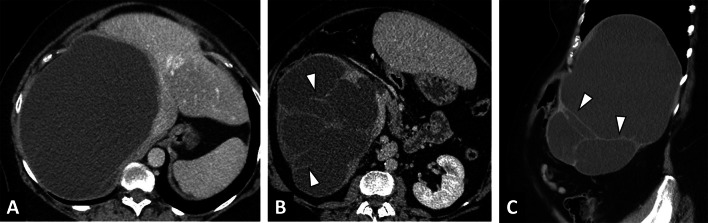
Differential diagnosis of cystic echinococcosis lesion in a 64-year-old woman. A CT image in the axial plane at the portal phase showing a large right liver cyst. B Same examination, lower slices. Internal enhanced septa. C CT image in the sagittal plane at the portal phase showing internal enhanced septa ruled out a cystic echinococcosis lesion, confirming the diagnosis of biliary mucinous cystadenoma
